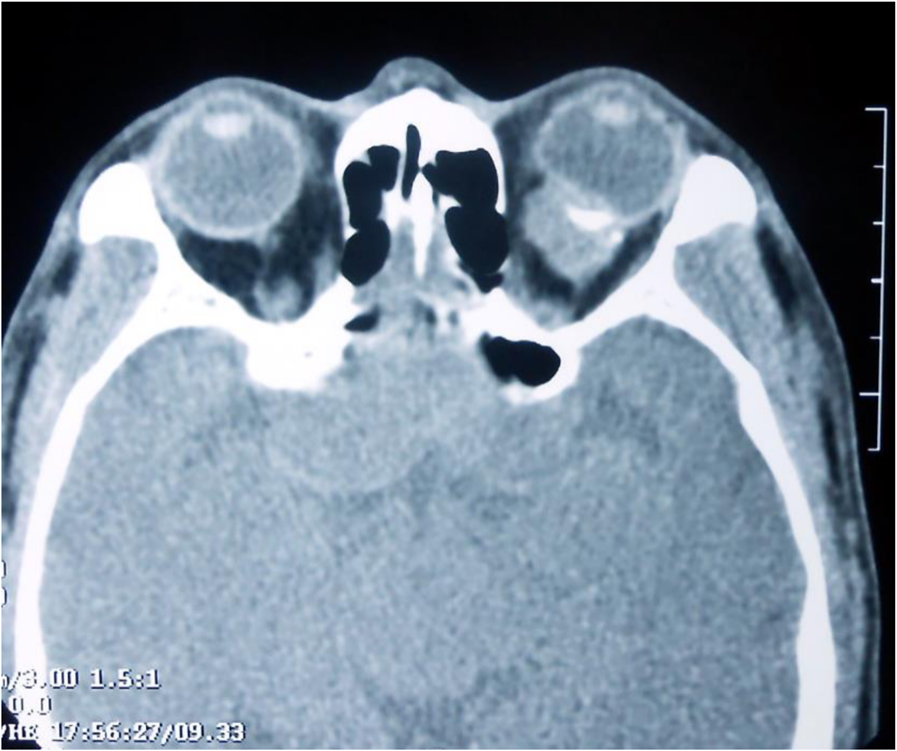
Preoperative CT in the recurrence
